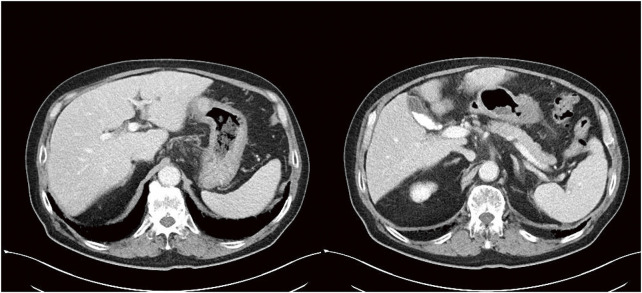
Contrast-enhanced CT findings after nivolumab combination chemotherapy. The stomach wall thickening had decreased, and the findings of peritoneal disseminated nodules and pancreatic infiltration had disappeared. CT, computed tomography
